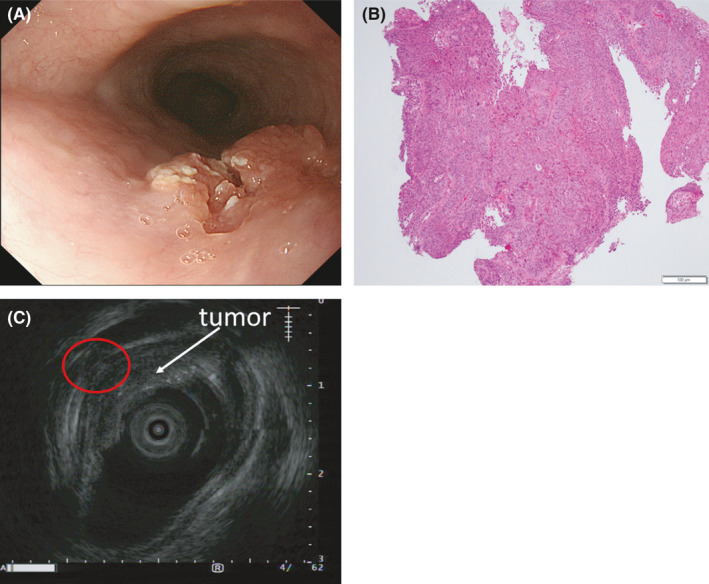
Esophageal findings at presentation (A) Esophagogastroduodenoscopy (EGD) reveals esophageal carcinoma 23 cm from the front teeth (B) A pathological picture of esophageal squamous cell carcinoma (C) Endoscopic ultrasound shows tumor invasion into the submucosal layer (red circle)
